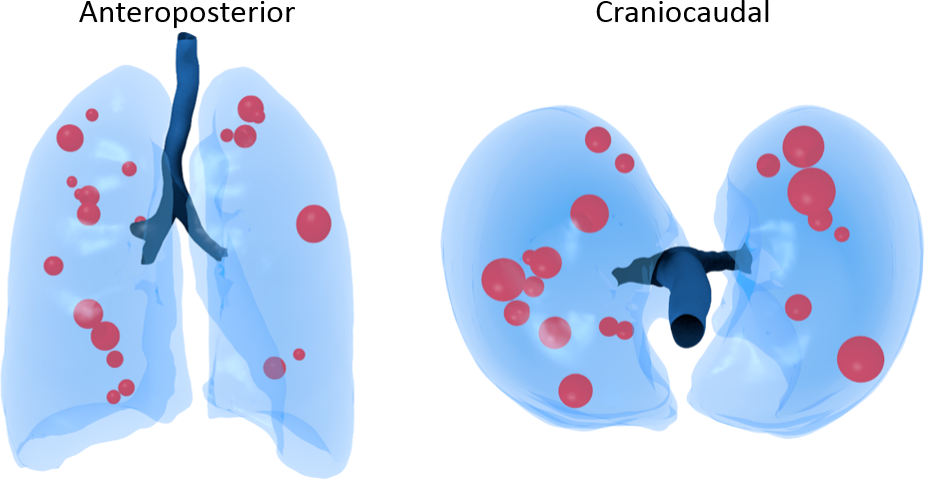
3D model of the lungs with the lesions (size and location) measured from the CT scans.
